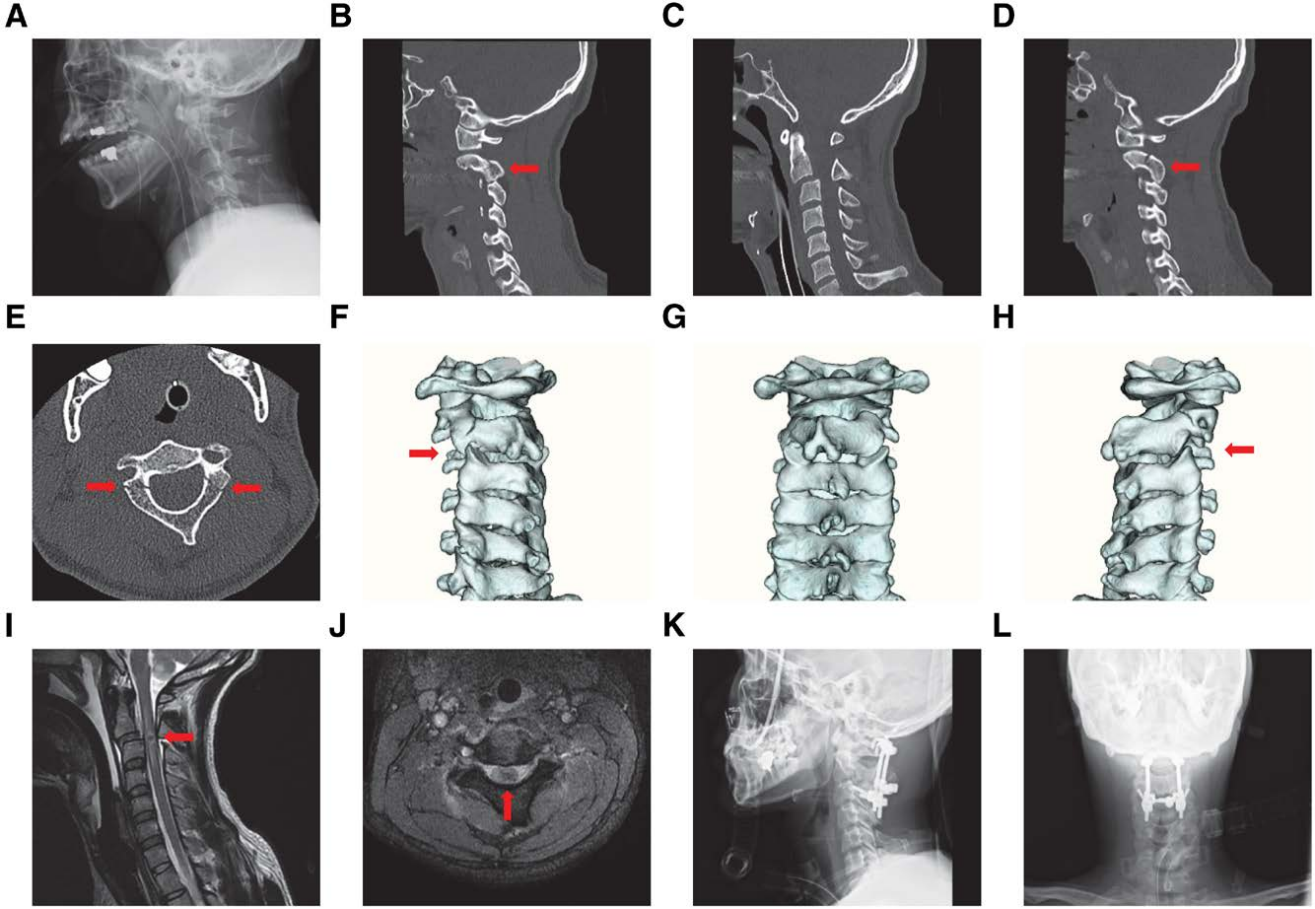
(A) Radiographic image of the patient at the time of injury. The patient suffered from severe tetraplegia with respiratory dysfunction and was intubated immediately after the injury. The image shows the C2 vertebral body displaced anteriorly. Computed tomography images of the cervical spine with C2 dislocated fracture in the sagittal section (B–D), axial section (E), and 3D reconstruction (F–H). Arrow heads show fracture of the left and right pars interarticularis of the axis. The left facet joint at C2–C3 was dislocated, and the superior articular process of C2 was perched on the lateral mass of C3 (B and F), whereas the facet joint at right C2–C3 was subluxated (D and H). MRI T2-weighted images of the patient at the time of injury in the sagittal section (I) and axial section (J) at the C2–C3 level. MRI images show a long segment lesion of T2-weighted hyperintensity signals around the injured level, with T2-weighted hypointensity signals at the epicenter. (K and L) Radiographic images of the patient after posterior cervical fusion 3 wk after the injury. MRI = magnetic resonance imaging.
